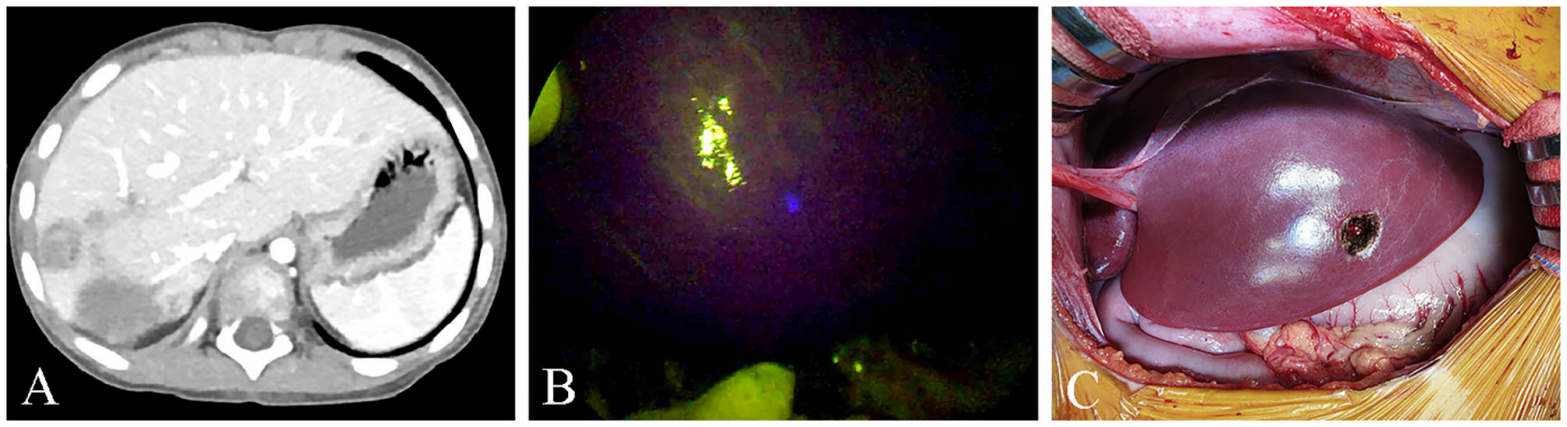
(A,B) One nodule was not seen on the pre-operative computed tomography (CT) but was seen on ICG/NIR camera. (C) Gross morphology.
